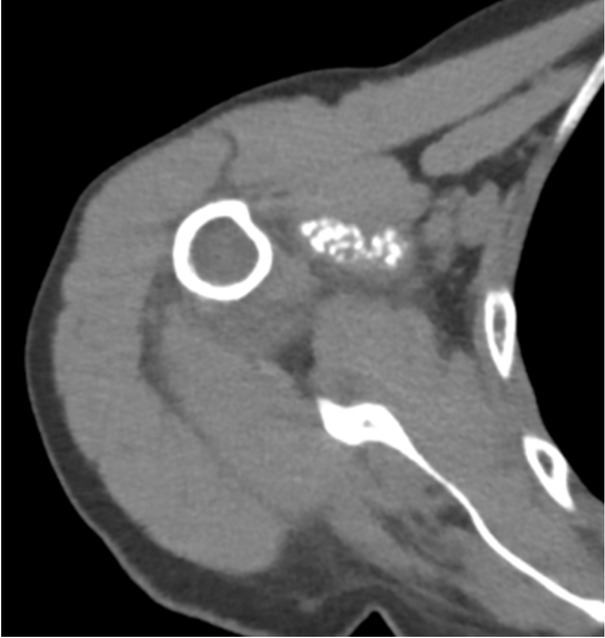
Computed tomography (CT) scan axial view showing the presence of calcifications within the supraspinatus muscle belly, an atypical location for Calcium pyrophosphate deposition (CPPD) deposits.
